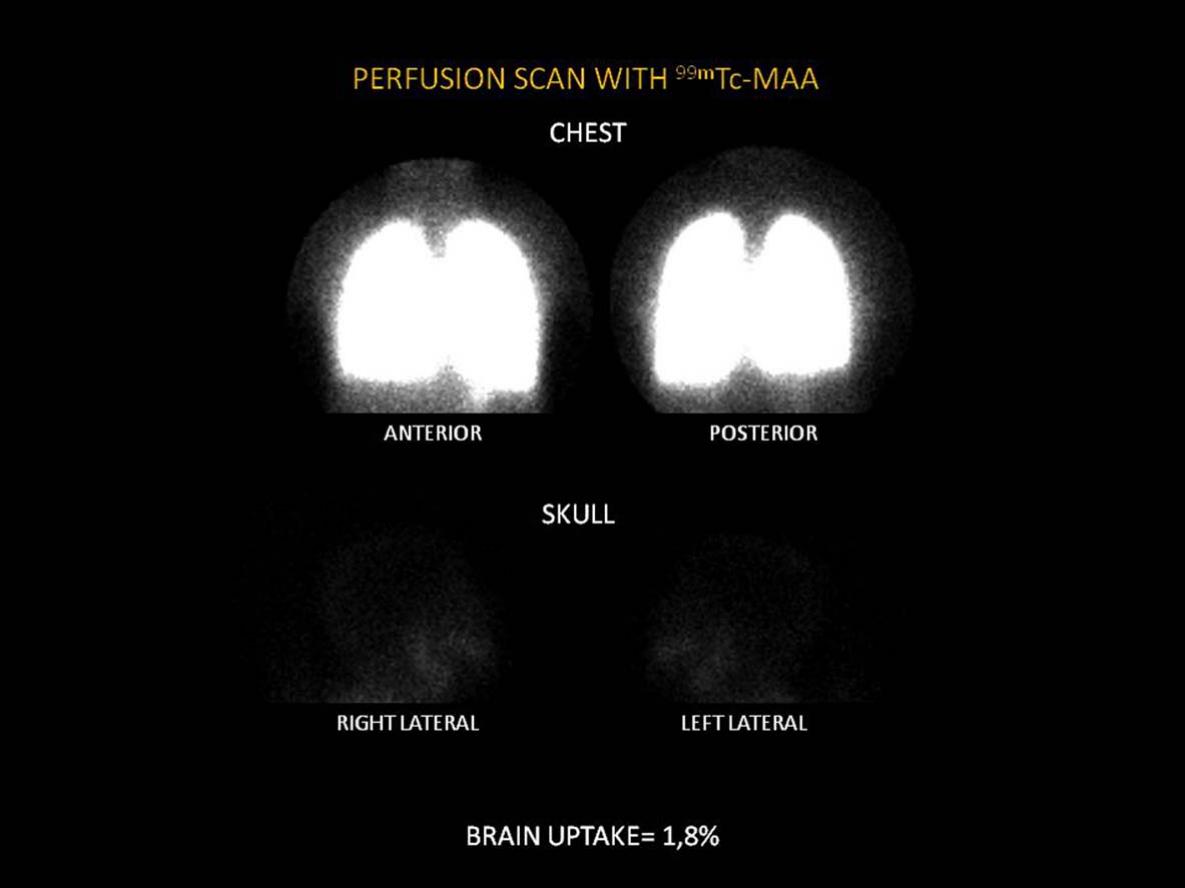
Normal scintigraphy. Brain uptake = 1.8%.
